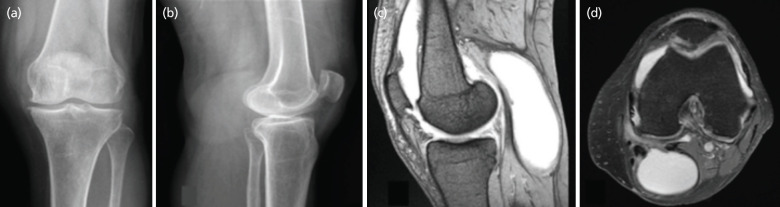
(a) Plain radiograph of the left knee AP, and (b) lateral view showing increased soft tissue density in the posterior part of knee. (c) Sagittal, and (d) axial view MRI showing Baker’s cyst extending between the semimembranous and medial head of gastrocnemius.
